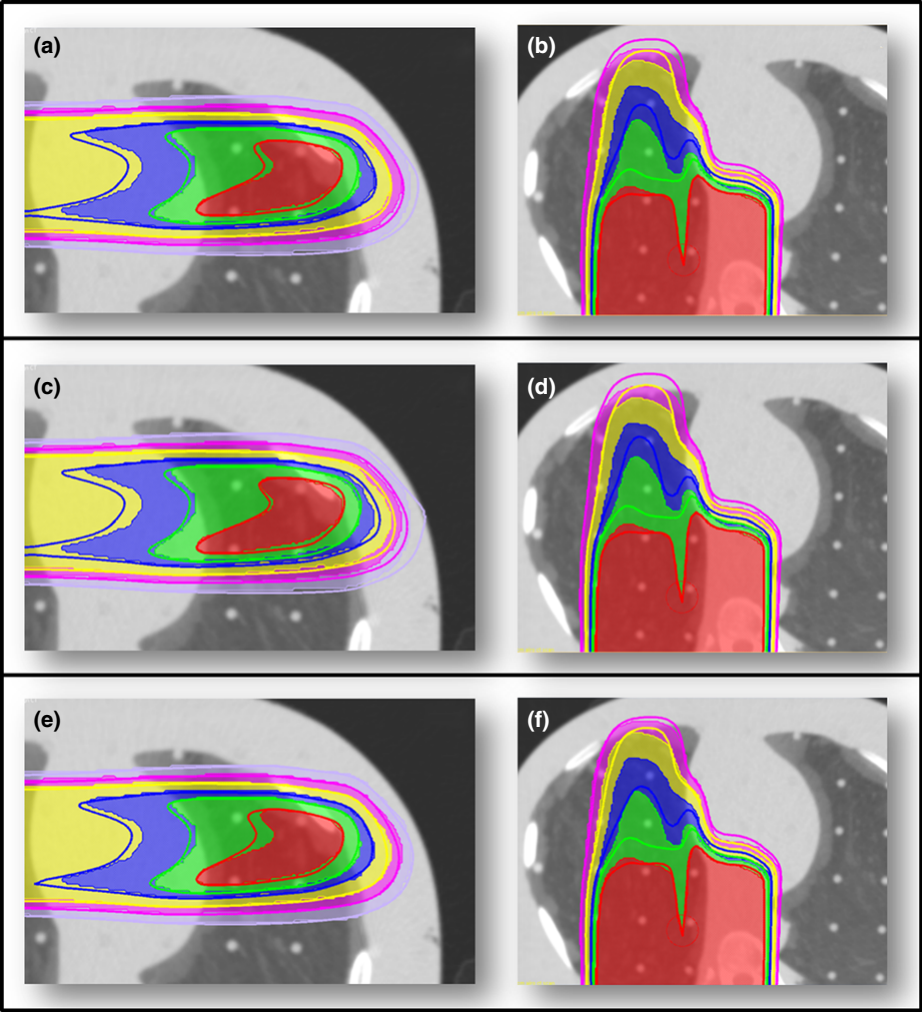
AIRO verification plans for RANDO Thorax AP and Lat plans: (a‐b) CT Sim SPR curve, (c‐d) AIRO Stoichiometric curve, and (e‐f) dose‐adjusted curve. The isodose with thin line represents the dose from the AIRO CT scan. The thick line represents the isodose lines from the original treatment planning CT. The red, green, blue, yellow, purple, and lavender lines represent: 20, 18, 16, 12, 8, and 4 Gy, respectively.
